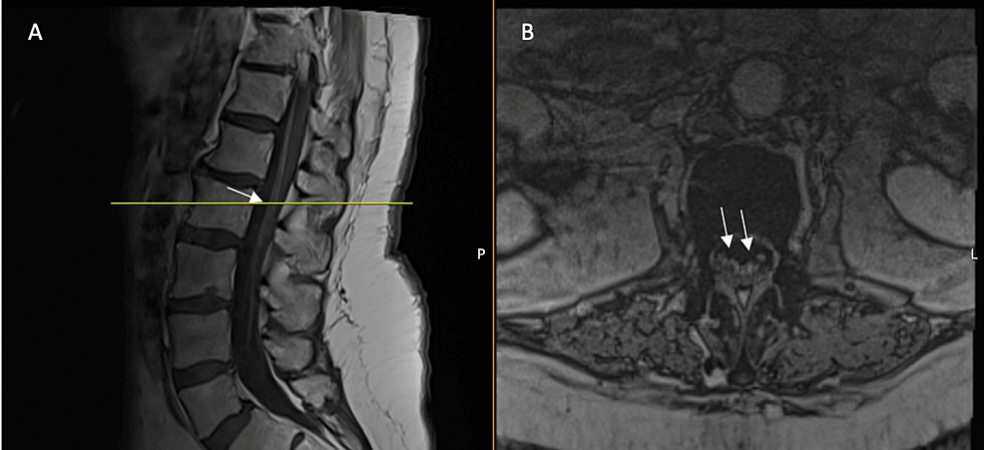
Contrast enhancement of the cauda equina nerve roots (arrows) on postcontrast T1-weighted lumbar spine MRI Sagittal (A) and axial (B) views. The horizontal line indicates the lumbar vertebral level MRI: magnetic resonance imaging
